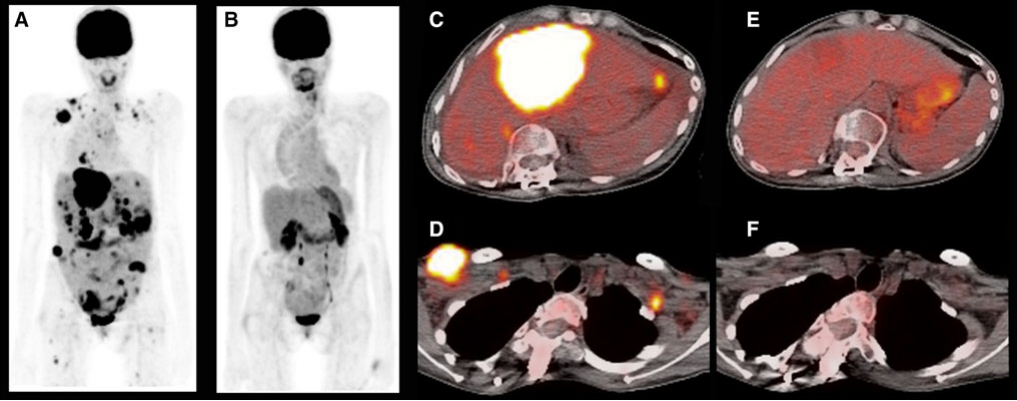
A 26-year-old female patient with metastatic melanoma with PET body maximum intensity projection images at the baseline (a) and after 8 weeks of treatment with a B-Raf inhibitor (b), demonstrating complete resolution of abnormal ^18^F-FDG uptake. Selected fused axial slices of a pretreatment and posttreatment hepatic lesion (c, e) and right chest wall lesion (d, f)
